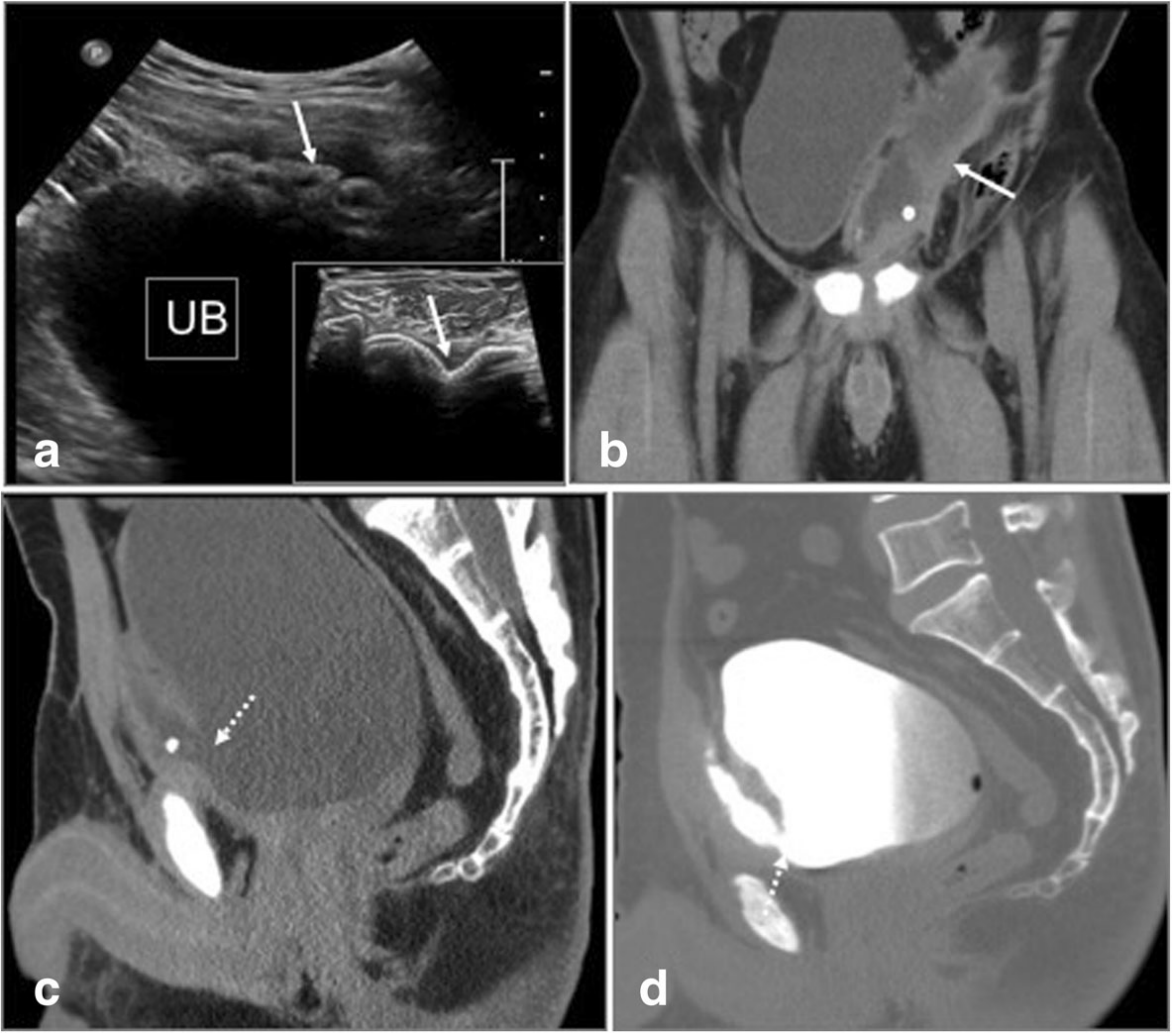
Post left inguinal hernia repair. a USG shows collection with migrated mesh (arrows) close to the urinary bladder (UB). b, c CT in coronal and sagittal planes show mesh eroding the anterior wall of urinary bladder (arrow) with fistulous communication (dashed arrow). d CT Cystogram confidently demonstrates the bladder communication (dashed arrow)
